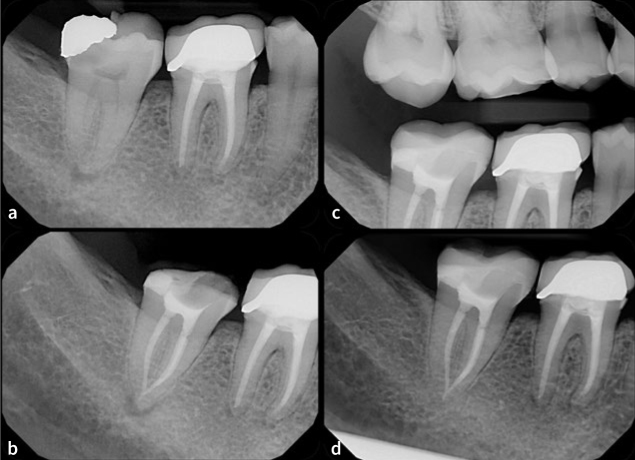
A periapical radiograph showing the mandibular right second molar with a defective disto-occlusal amalgam restoration, recurrent caries and a radiolucent apical lesion (a). A diagnosis of necrotic pulp with asymptomatic apical periodontitis was made. Root canal treatment and deep marginal elevation of the distal margin were performed (b). Following a follow-up period of 23 months, a bitewing (c) and periapical (d) radiographs show good adaptation of the DME material and the direct restoration, and the resolution of the apical pathology.
